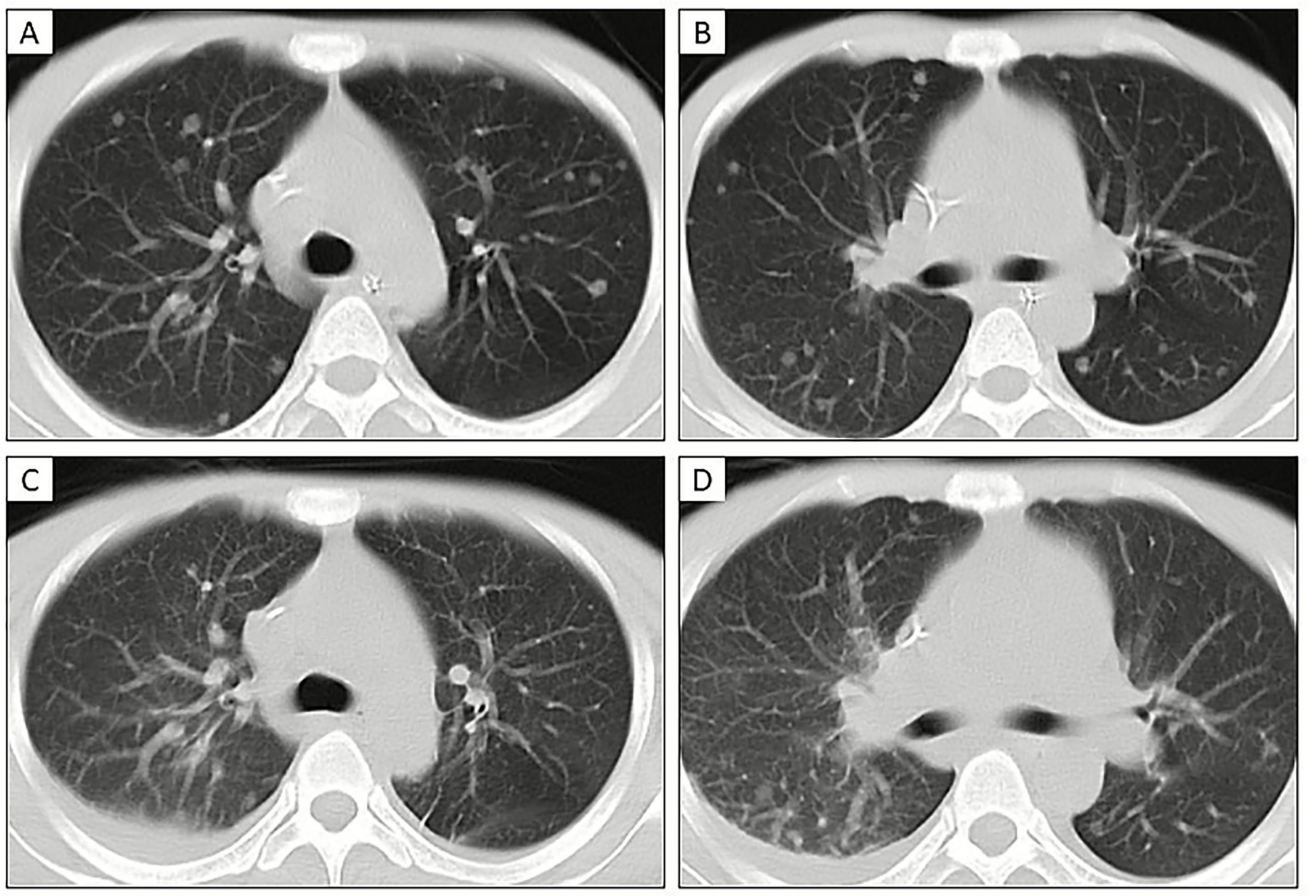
24-year-old man with pneumonia caused by Mpox who had genital skin rashes as initial symptoms. (A,B) CT axial scans show bilateral pulmonary solid nodular shadows; (C,D) following ART treatment, CT scans on October 9 show a reduction in the number of nodules, decreased density, and diminished ground-glass nodular shadows, with pleural effusion on the right side.
